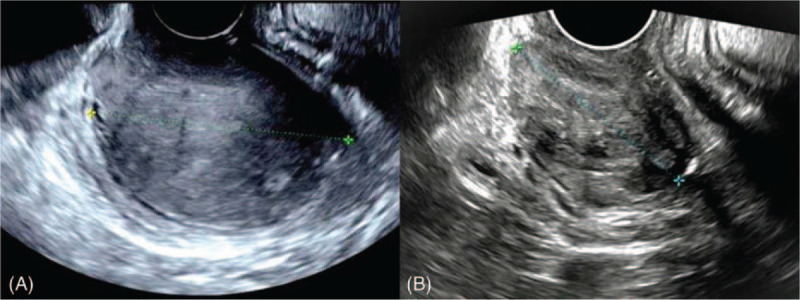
Transvaginal ultrasound showed a hypo-echoic mass on the anterior vaginal wall before (A) and after (B) treatment of GnRH analogue.
